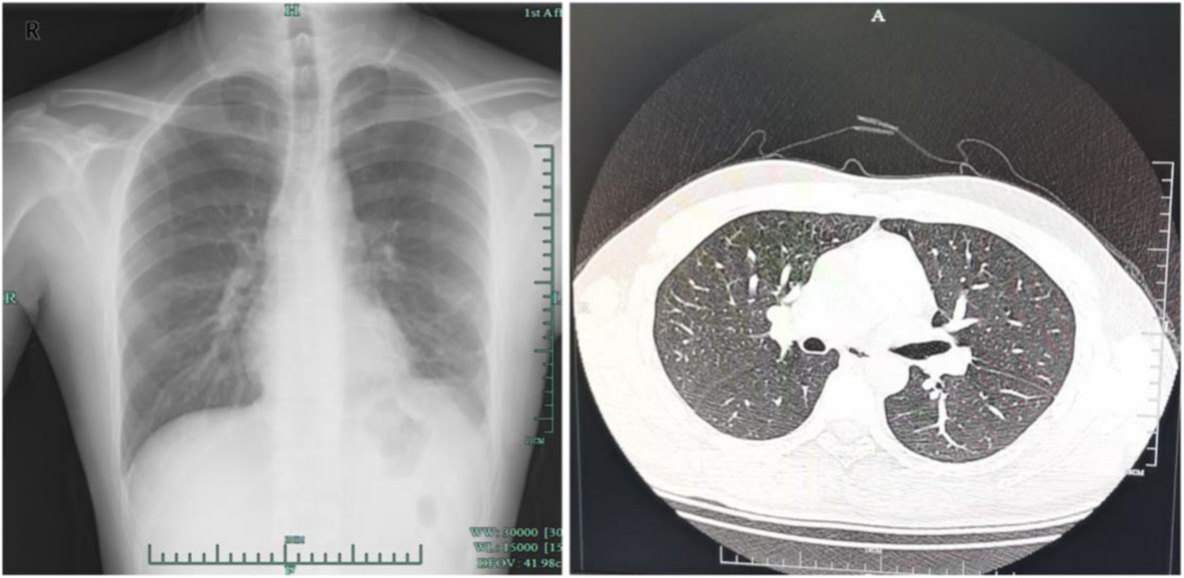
Chest X ray and CT scan of the patient 8 months after the surgery, which showed no evidence of recurrence
